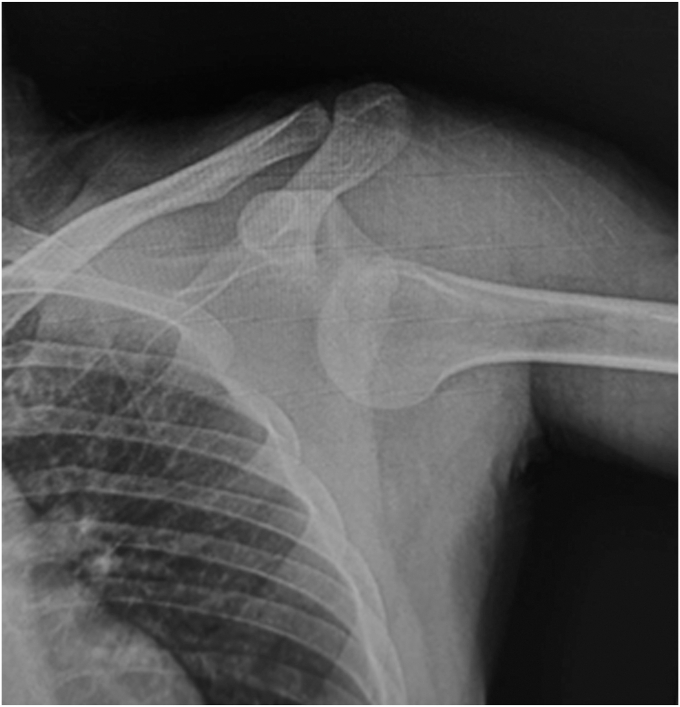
Radiograph of the left shoulder acquired on the day of polytrauma, demonstrating anteroinferior glenohumeral dislocation.
